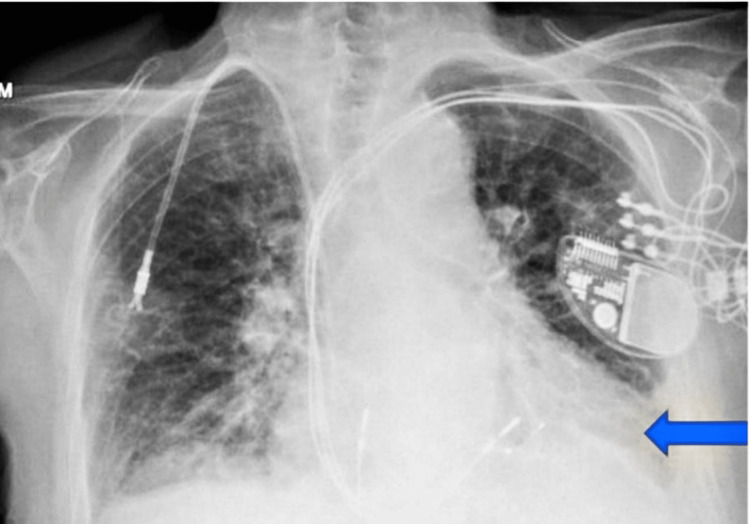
Chest x-ray showing pleural effusion (blue arrow)
